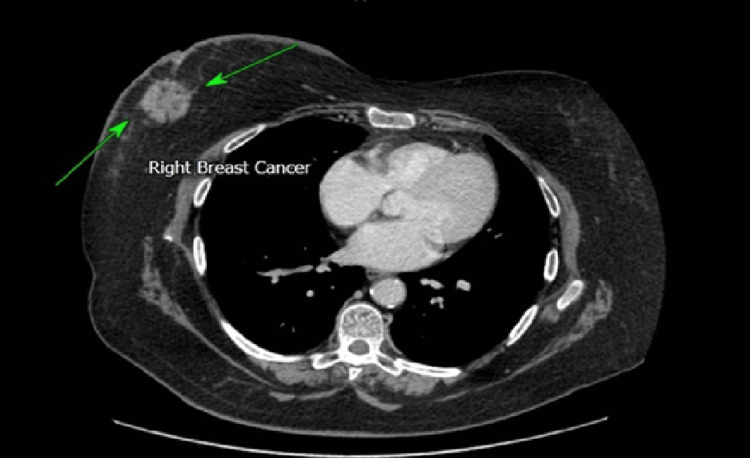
(Case 1) Axial plane contrast-enhanced CT image shows evidence of right breast carcinoma (Green arrows) associated with overlying skin thickening.
